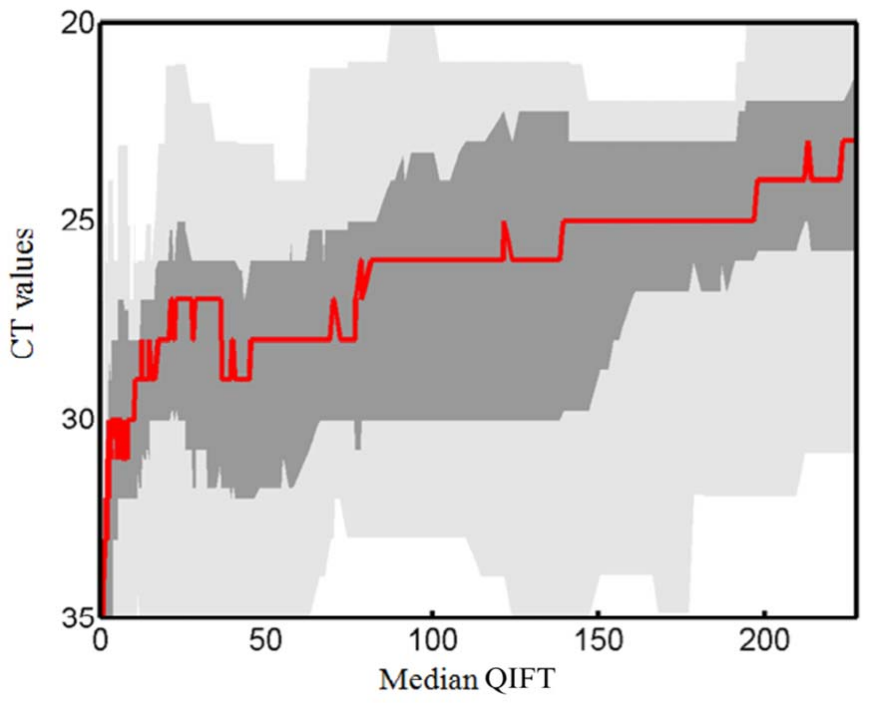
Sliding Window Graph: Binning of median CT values and ranges/percentiles based on median QIFT.
